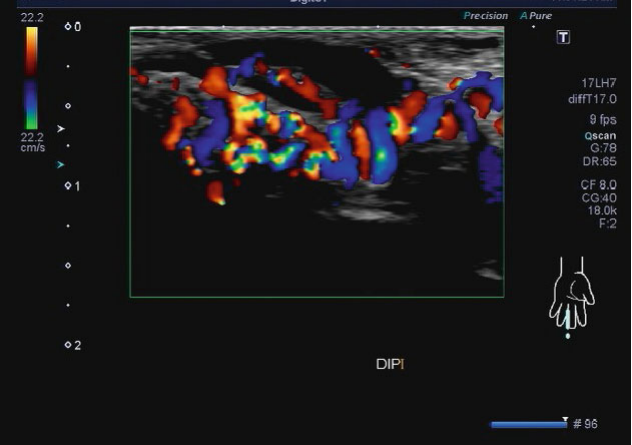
Ultrasound examination image of the ulnar side of the left swollen middle finger. Numerous tortuous dilated vessels with chaotic mosaic-like multidirectional flow were noted from the base of the left middle finger to the distal phalanx.
